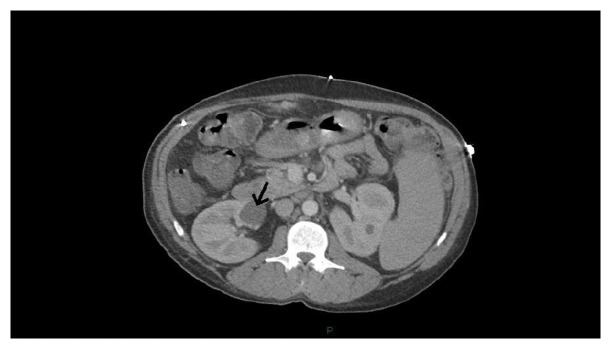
Axial image from CT of the abdomen with IV contrast obtained 65 days prior to admission revealed a simple right renal cyst (black arrow) in the location of the now infected cyst.
